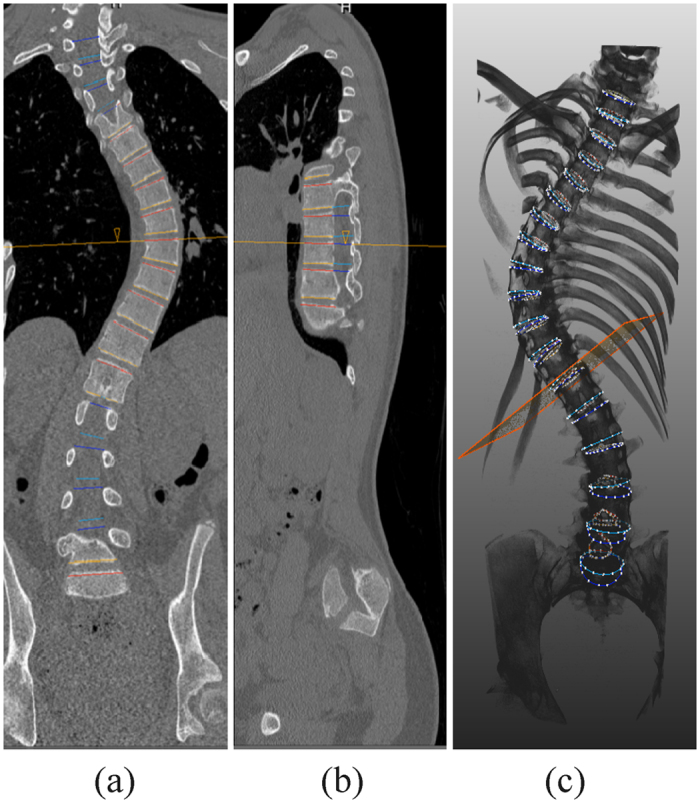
Example of semi-automatic analysis of the vertebrae and intervertebral discs on high resolution CT scans. (a,b) Represent the delineation of the upper/lower endplates in sagittal and coronal planes, respectively. (c) An example of a complete 3-D reconstruction and coordinate system based on the delineation and segmentation.
